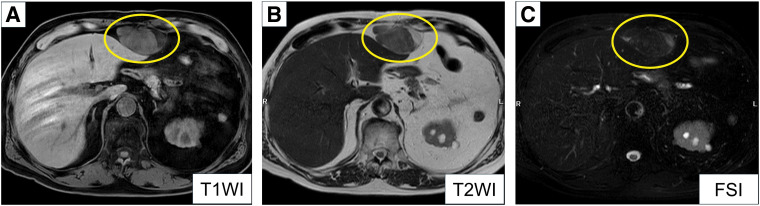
MRI findings. (A) T1WI demonstrated a lesion with mixed low- and high-signal intensities (yellow circle). (B) T2WI demonstrated a lesion with mixed low- and high-signal intensities (yellow circle). (C) On FSI, the signal intensity of the lesion decreased, indicating the presence of adipose tissue (yellow circle). FSI, fat-suppression image; T1WI, T1-weighted image; T2WI, T2-weighted image
